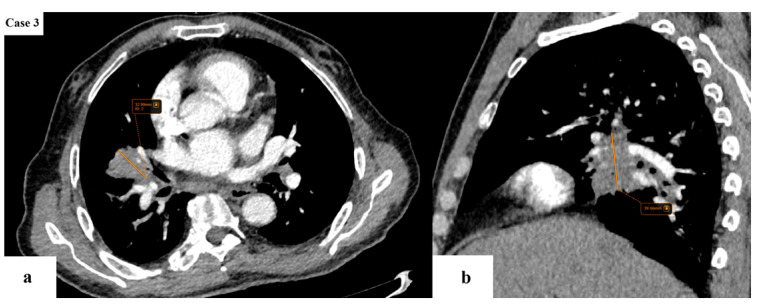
Axial (a) and sagittal (b) CT reconstructions of a 39 mm central lung mass located in the lateral segment of the right middle lobe, with extension into the superior segments of the right lower lobe.
